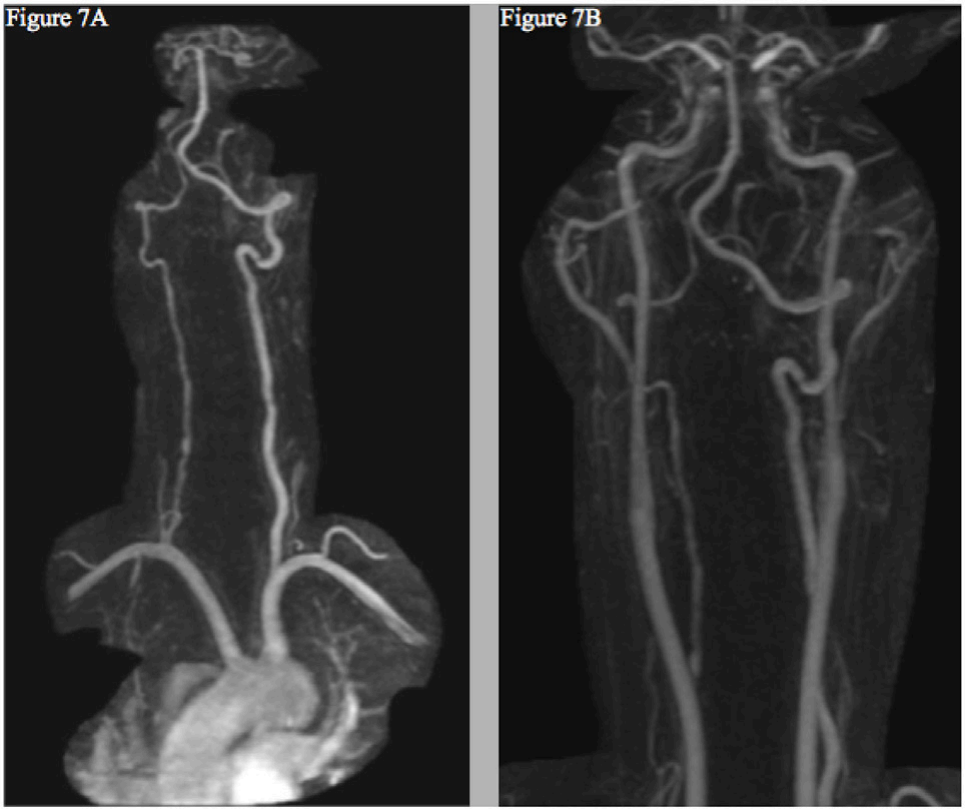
Follow-up MRI angiogram showing interval improvement of the left vertebral (A) and internal carotid arteries (B)—without evidence of new dissection or aneurysm—and persistent right cervical vertebral artery contour irregularity (A).
